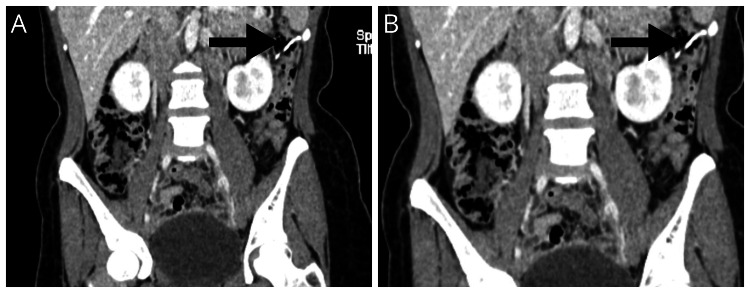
CT of the abdomen coronal section showing the IUCD in the descending colon (arrow) CT: computed tomography, IUCD: intrauterine contraceptive device
